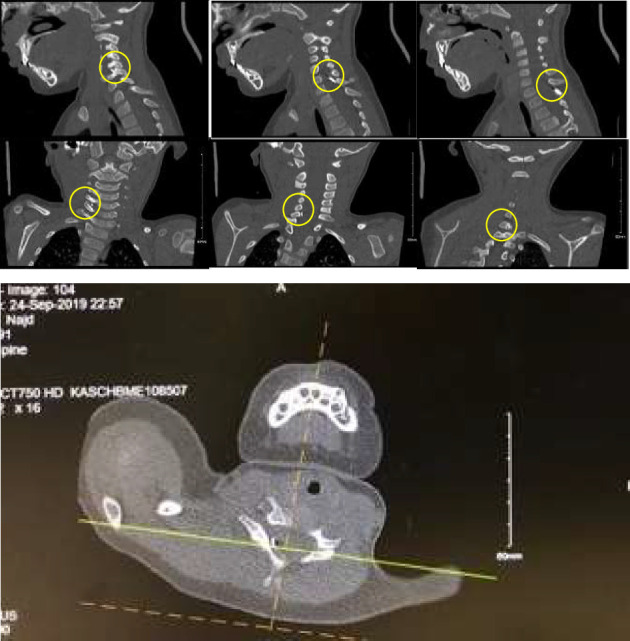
CT cervical with sagittal, coronal, and axial views showing an obliquely oriented hyperdense linear foreign body, likely a needle, measuring about 4 cm and starting from C7-T1 interspinous space close to the left lamina of T1 extending superiorly to the right and ending at the C5-C6 level, abutting the C5-C6 exiting right nerve root as well as the foraminal segment of the right vertebral artery (VA).
